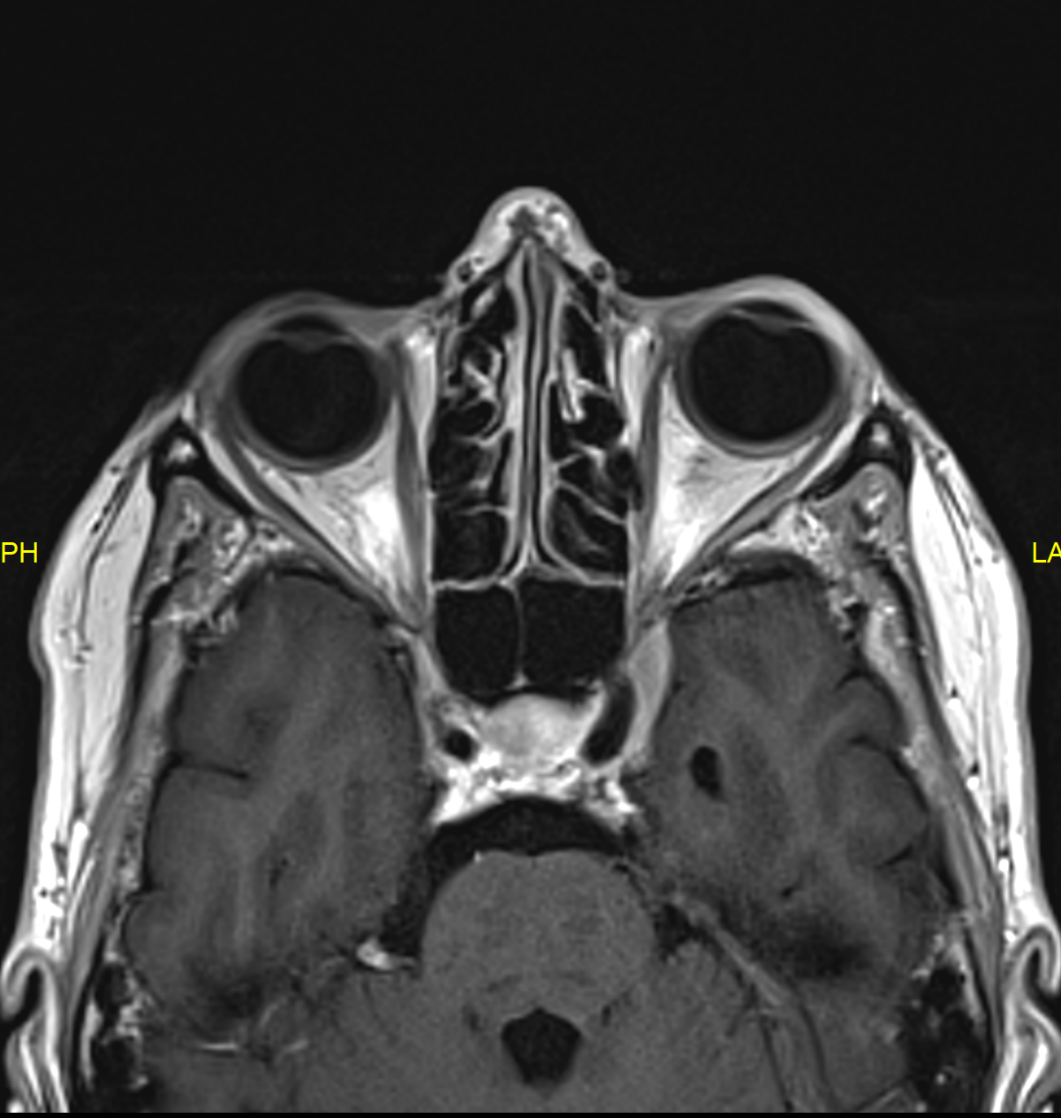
MRI scan (2017) showing dural enhancement at the left cavernous sinus
